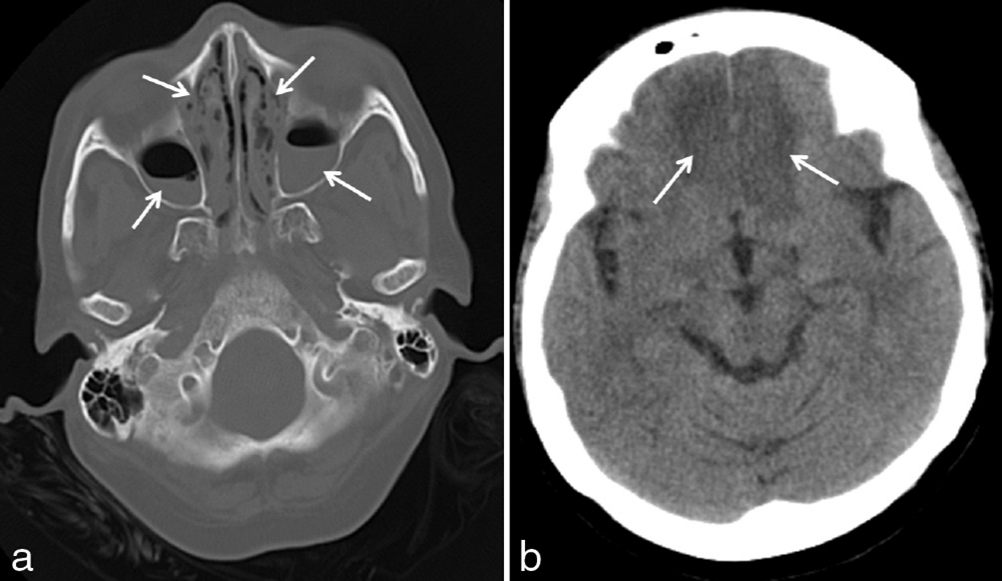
(a) Axial plain CT scan in bone window after 5 days showing worsening sinusitis with development of air–fluid levels in bilateral maxillary sinuses (arrows). (b) Plain CT scan in the axial section at the level of the brain showing interval appearance of hypodense areas in bilateral inferior frontal lobes (arrows).
